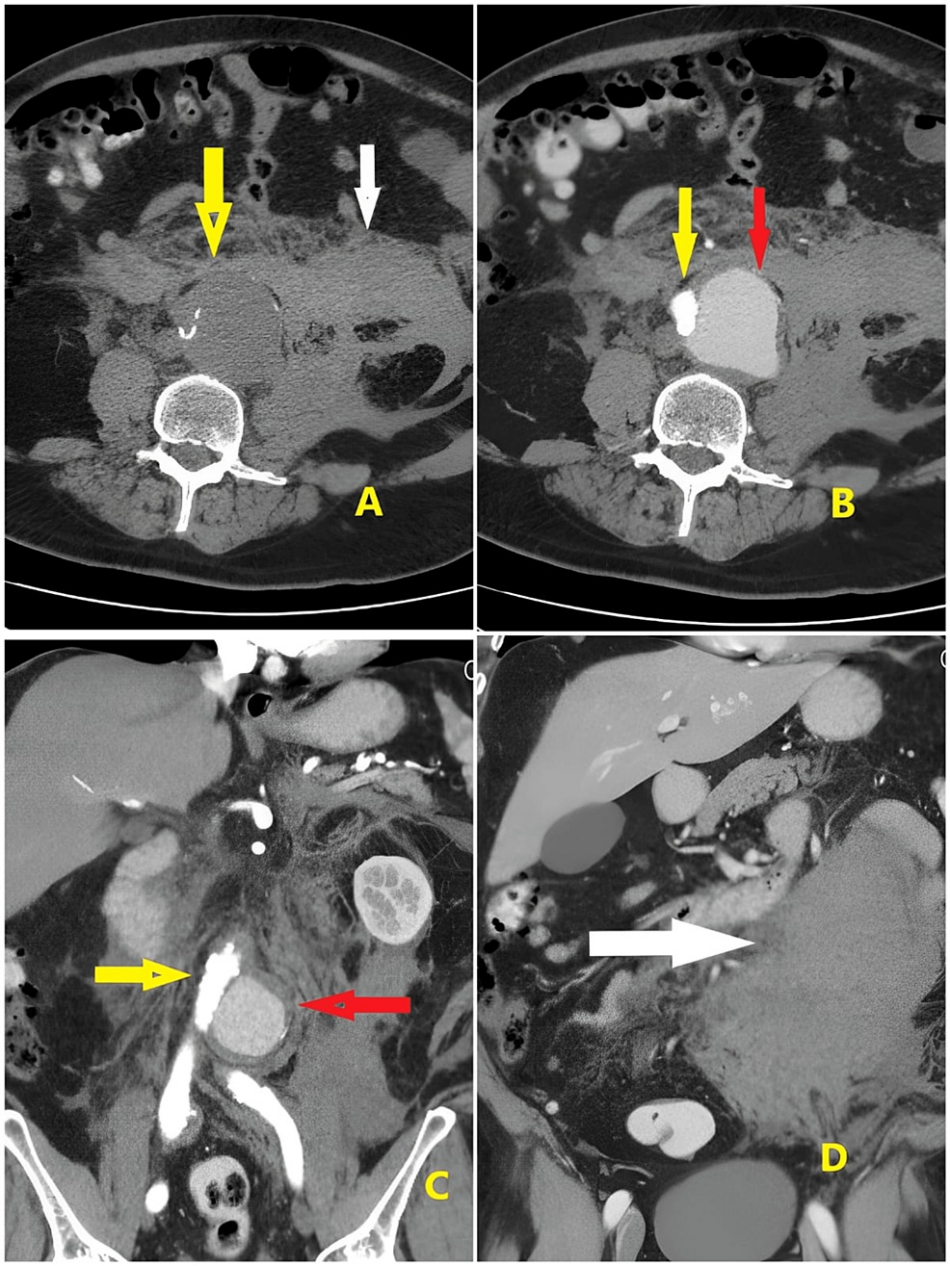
(A) Plain CT of the aorta without contrast (yellow arrow) and surrounding hematoma (white arrow), (B and C) the true lumen (yellow arrow) and the false lumen (red arrow), and (D) a huge retroperitoneal hematoma (white arrow) CT: computed tomography
